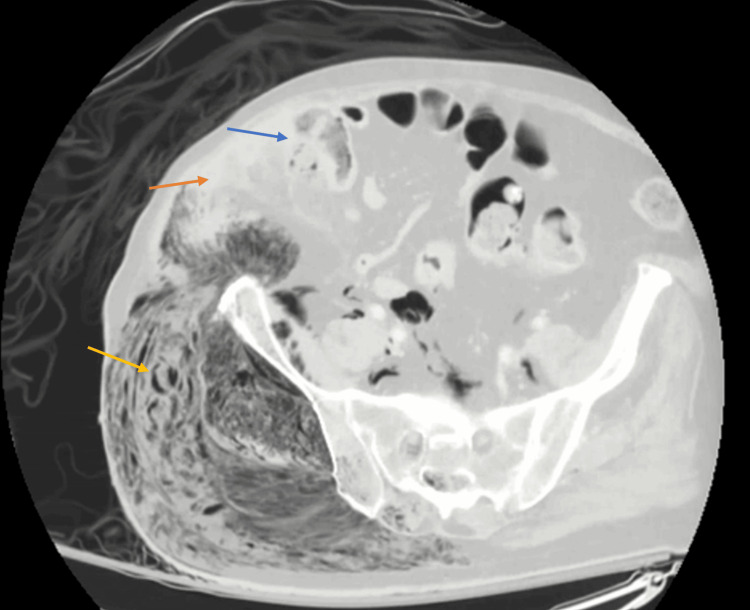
CT of the abdomen and pelvis revealing a cecal mass with micro-perforation The blue arrow shows the cecal mass and site of micro-perforation. The orange arrow shows the fluid tracking laterally out of the cecum. The yellow arrow shows the necrotizing infection and gas CT: computed tomography
